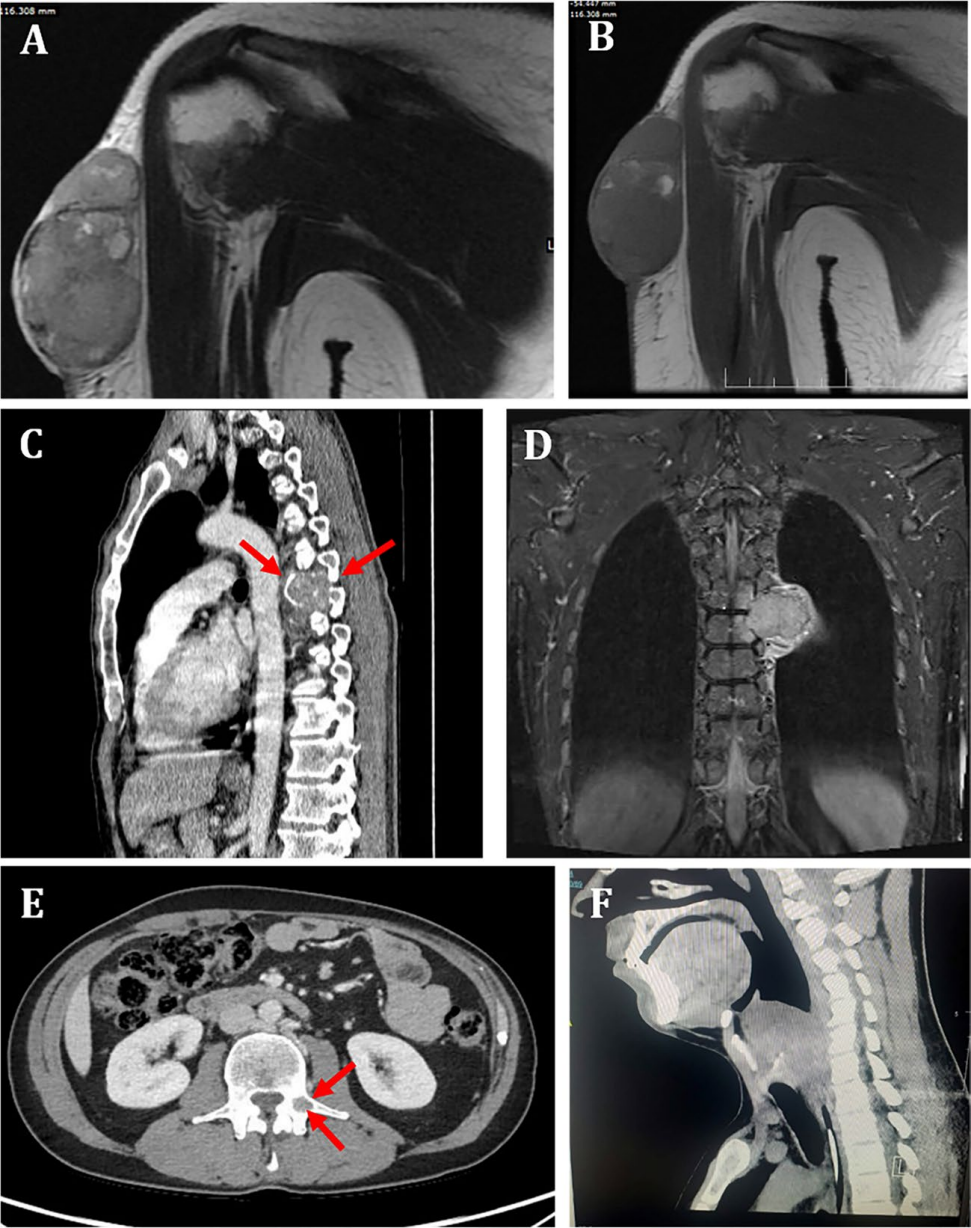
Representative imaging findings. A and B (Case 1): large, well-circumscribed, lobulated mass, isointense to muscles on T1 and heterogeneously hyperintense on T2-weighted images, measuring 7 cm cranio-caudally. C (CT) and D (MRI) in Case 2 revealed a large destructive mass (arrows) likely originating from the 7th rib and invading into the 6th and 7th thoracic vertebral bodies without intraspinal extension. E Progressive osteolytic metastases in same patient were detected in 2nd and 3rd lumbar vertebrae on follow up and verified by core needle biopsies (arrows). F CT of the head and neck in Case 4 revealed large neck soft tissue mass adjacent to the supraglottic larynx and the paralaryngeal tissue
